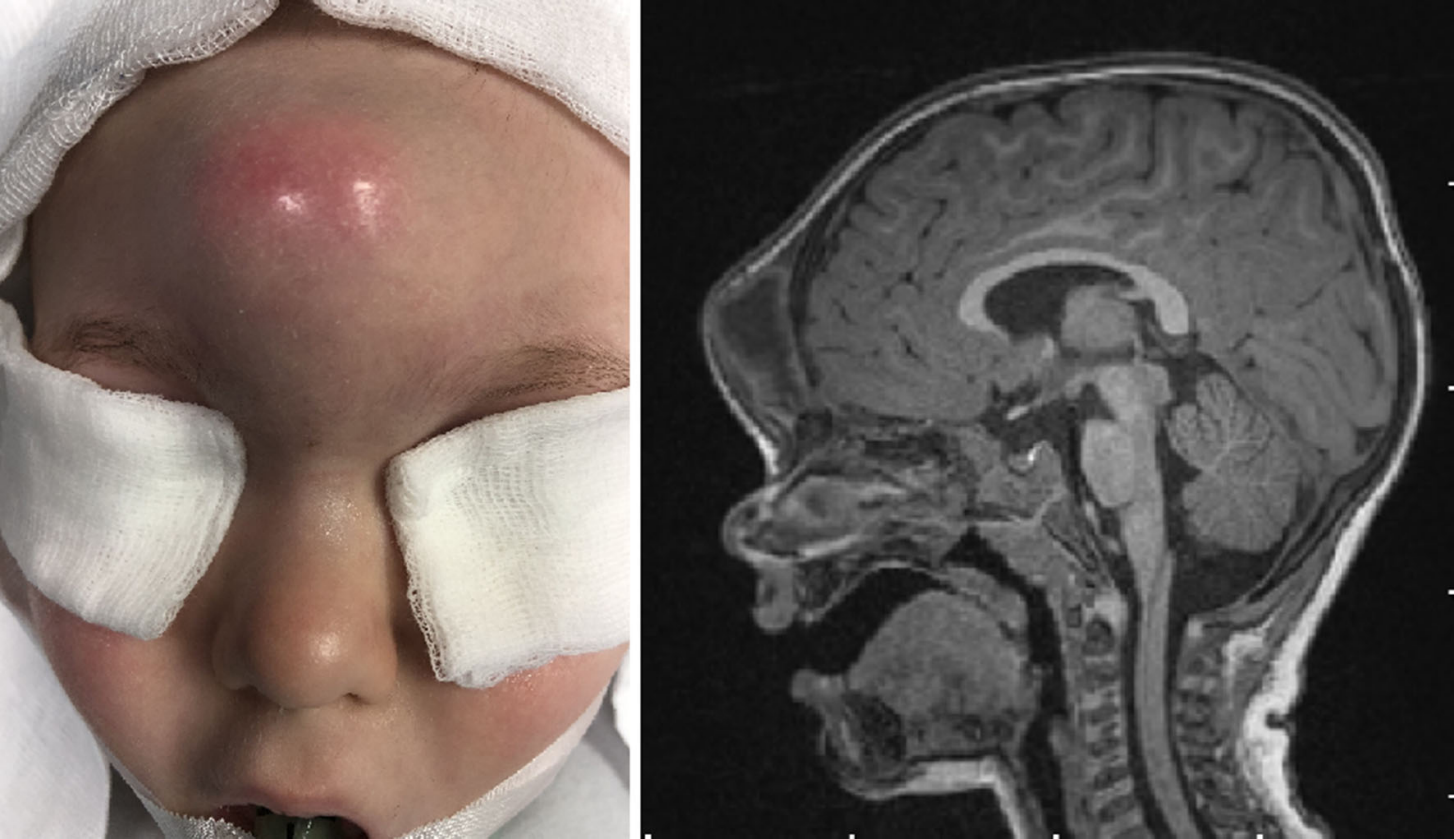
Complication of the nasal dermoid in 18-month-old male patient. A massive abscess of the frontal region—clinical manifestation and MRI-T1 sagittal view
